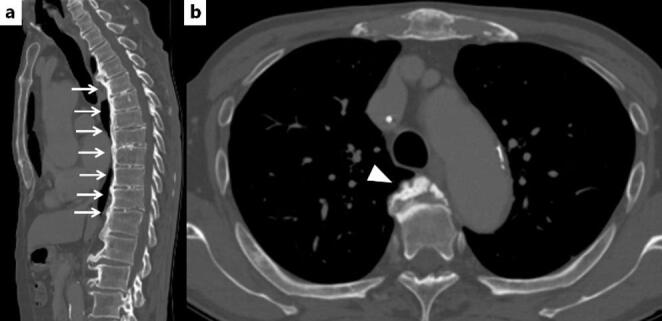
Diffuse idiopathic skeletal hyperostosis (DISH). a sagittal CT (bone window), b axial CT (bone window). A man in his 70s with asymptomatic type 2 DM. Sagittal CT (a) shows flowing anterior ossification bridging multiple contiguous vertebral bodies (arrow) with relative preservation of disc height. Axial CT (b) at the corresponding level demonstrates bulky anterolateral ossification (arrowhead). Abbreviations: CT, computed tomography; DM, diabetes mellitus
